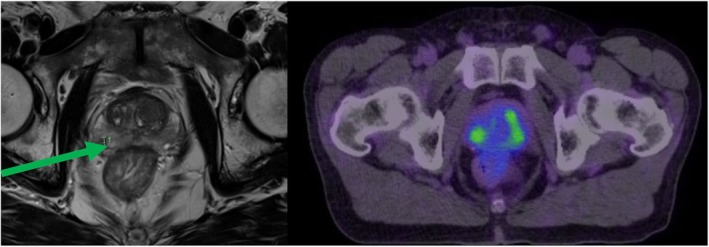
Example of missed outfield lesion, with focal lesion identified on T2 MRI imaging at right posterolateral peripheral zone, compared to multifocal PSMA uptake on PSMA PET.
